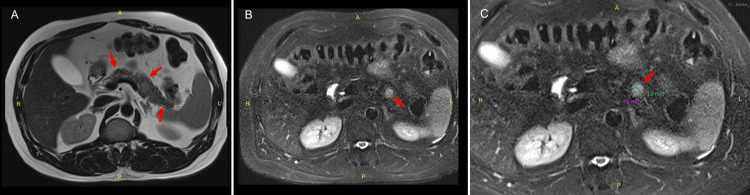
MRI demonstrating a pancreatic body lesion consistent with insulinoma. (A) Axial T2-weighted image without a clearly identifiable focal lesion (red arrows). (B) Axial STIR image demonstrating a focal lesion in the pancreatic body (red arrow). (C) Magnified STIR image showing a well-defined pancreatic body lesion measuring 16 × 13 mm (red arrow). MRI, magnetic resonance imaging; STIR, short tau inversion recovery. Orientation markers: A, anterior; P, posterior; R, right; L, left.
